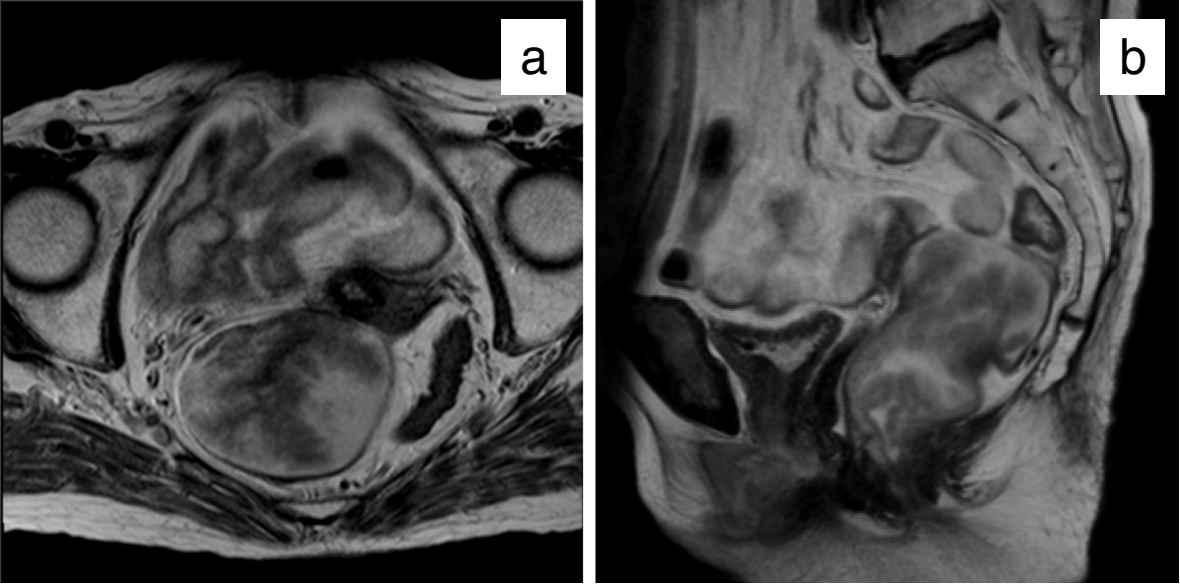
Magnetic resonance imaging findings. a Axial. b Sagittal. There was no sign of invasion up to the vagina and anal sphincter
